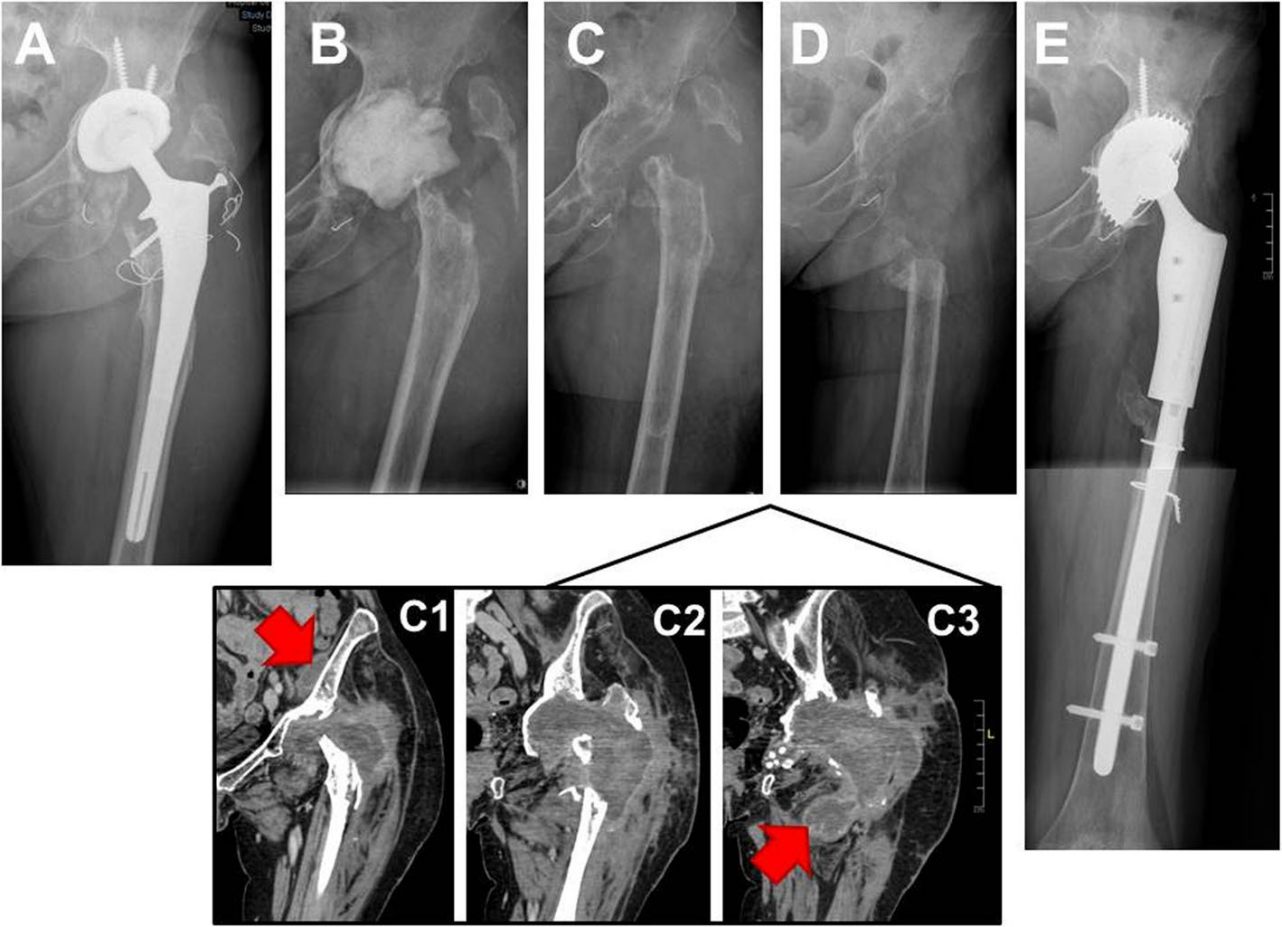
X-ray and CT-scan of the left hip performed: A at admission; B after prosthesis explantation, debridement and set-up of a spacer; C after performance of a new debridement and spacer removal, unfortunately with recurring abscesses visible on CT-scan (C1: ilio-psoas recurrent abscess, red arrow; C2: large collection within the joint; C3: abscesses in the thigh, red arrow) despite prolonged hydroxychloroquine-doxycycline treatment; D after a subsequent surgery with debridement of the recurrent abscesses and performance of a femoral resection; E 6 years after reimplantation of a megaprosthesis, no prosthesis loosening
